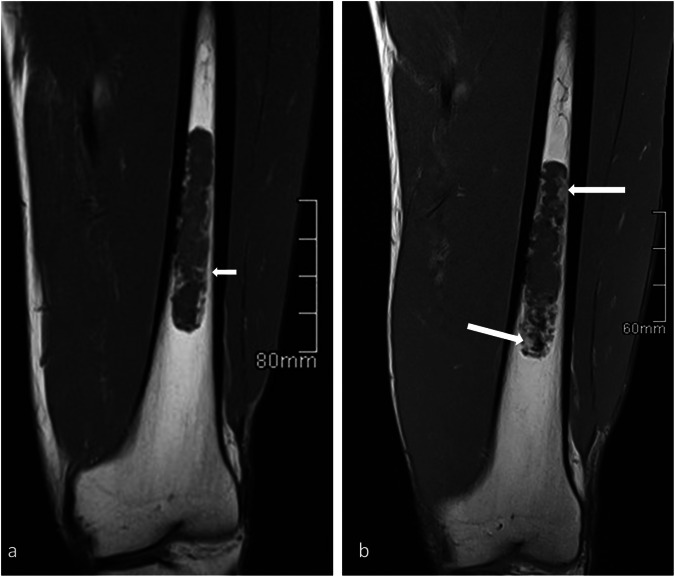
a TSE T1-weighted coronal baseline MRI showing small spots of fat entrapment (small arrow) in an LG-CCL and (b) follow-up MRI 6.5 years later; arrows pointing at fat replacement. LG-CCL, Low-grade central cartilaginous lesion
